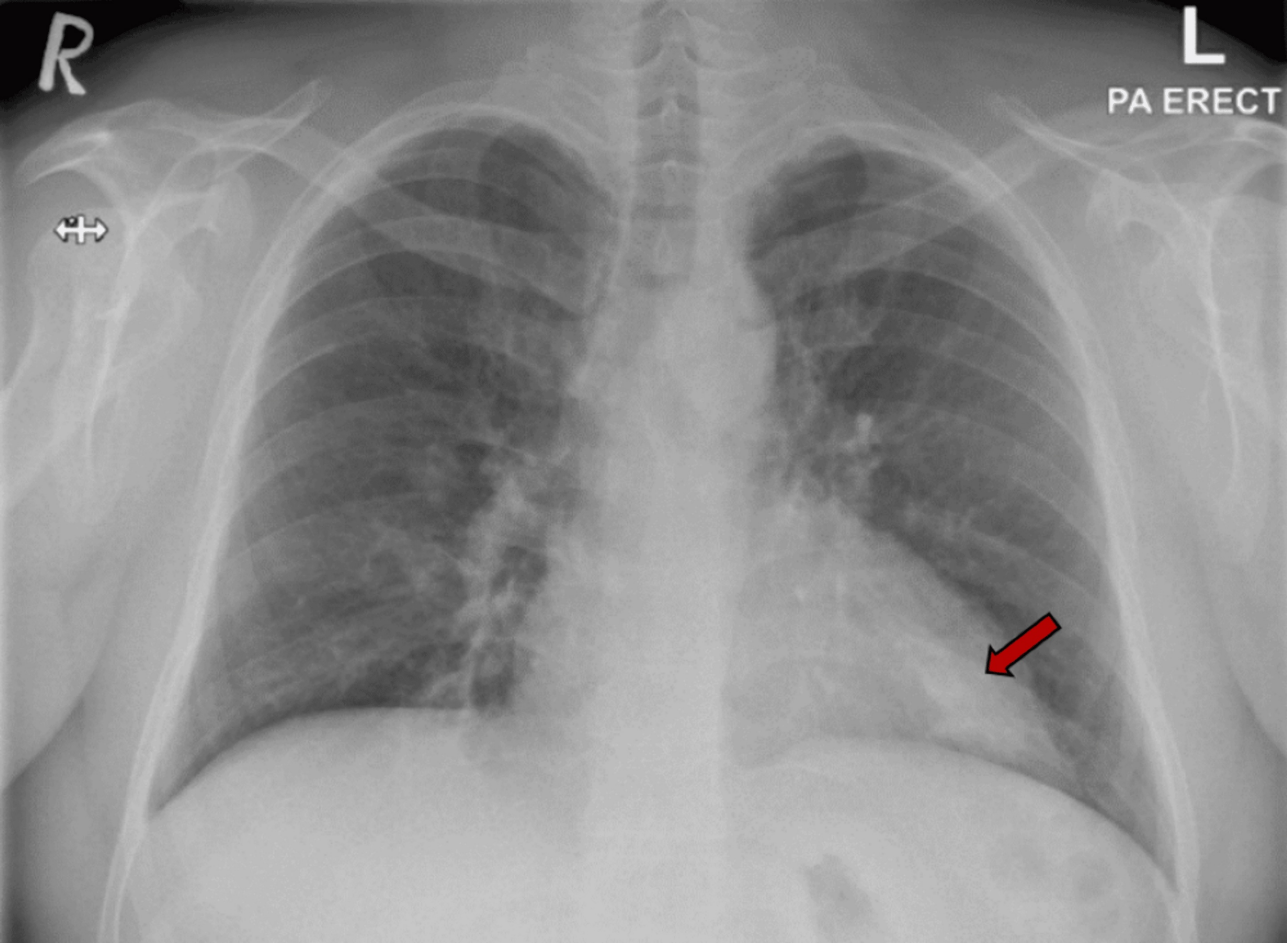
The posteroanterior chest radiograph shows a left retrocardiac patchy consolidation (arrow).
